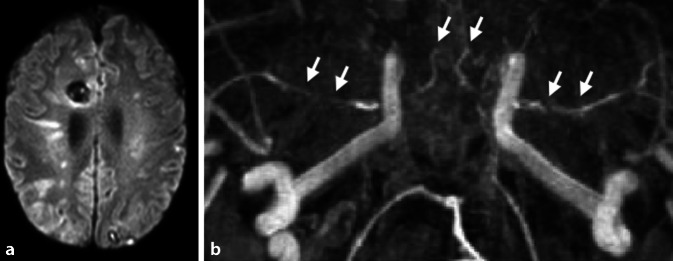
Magnetresonanztomographische Aufnahmen eines Patienten mit bakterieller Meningitis infolge einer Mastoiditis rechts. a Die DWI(„diffusion weighted imaging“)-Wichtung zeigt multiple zerebrale Ischämien im vorderen und hinteren Stromgebiet sowie putrides Sediment, hauptsächlich innerhalb der rechten Hemisphäre mit räumlichem Bezug zur Mastoiditis auf dieser Seite. b In der TOF(„time-of-flight“)-Angiographie sind multiple Verengungen der intraduralen Abschnitte der hirnversorgenden Gefäße als Ausdruck einer Vaskulopathie nachweisbar (*Pfeile*)
